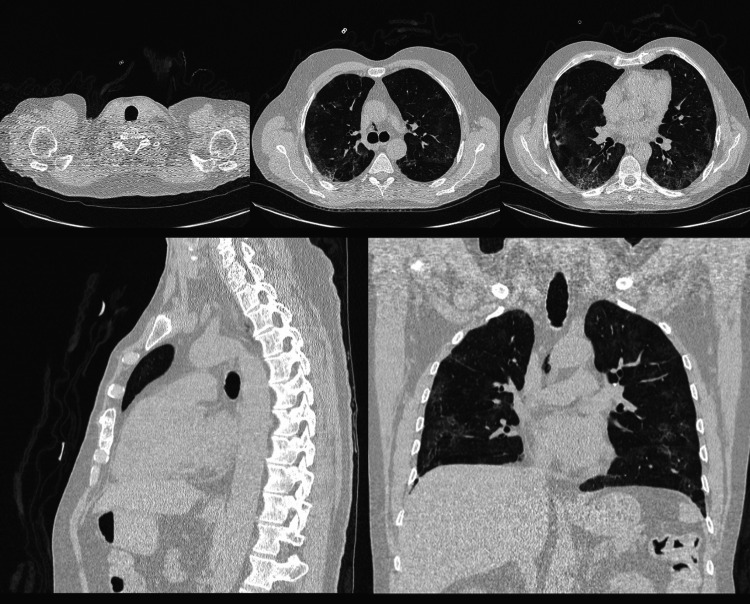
CT scan showing complete resolution of pneumomediastinum and subcutaneous emphysema.
